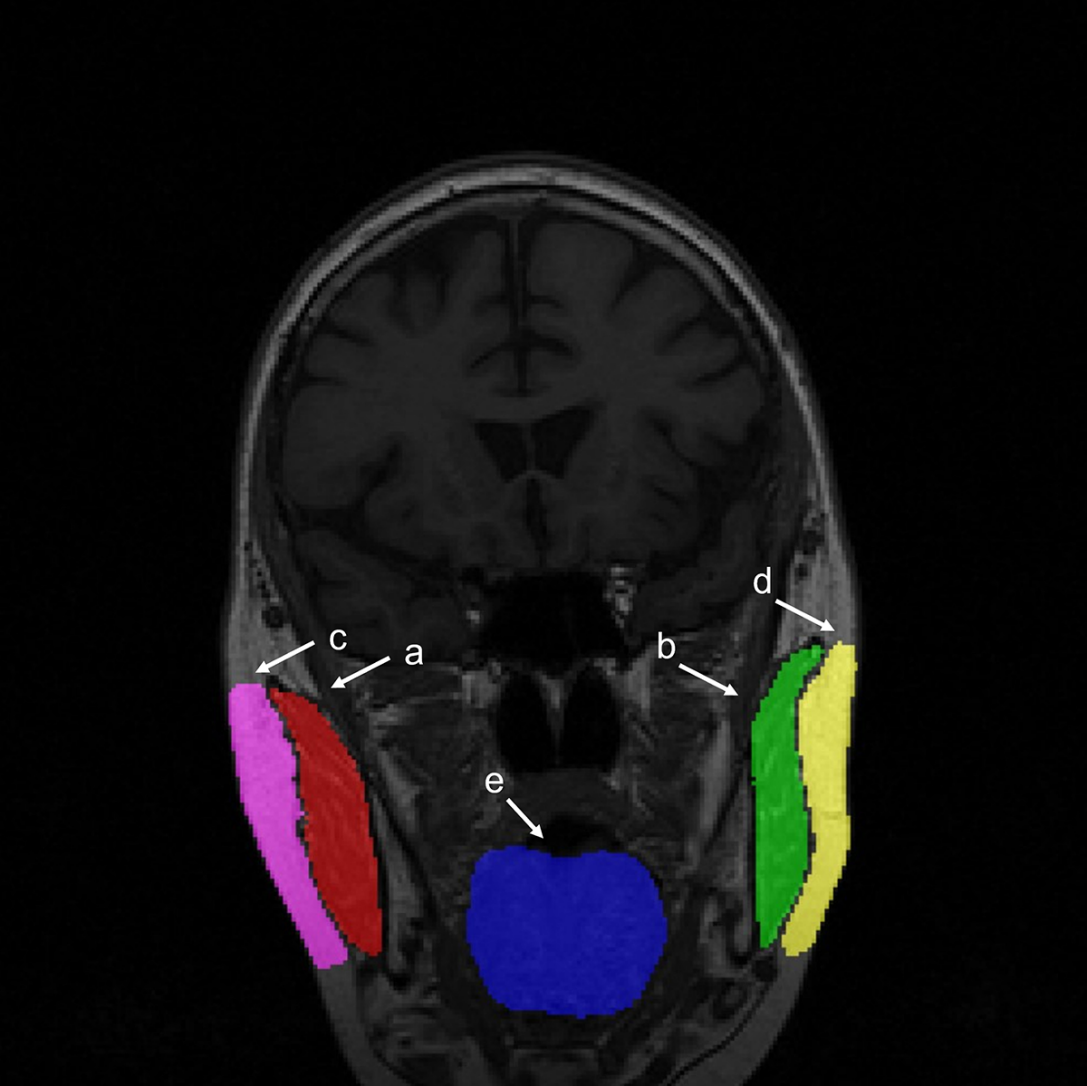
Example of segmented tissues overlaid on the original MRI. (A) Right masseter muscle, (B) left masseter muscle, (C) right subcutaneous fat, (D) left subcutaneous fat, and (E) tongue muscle.
